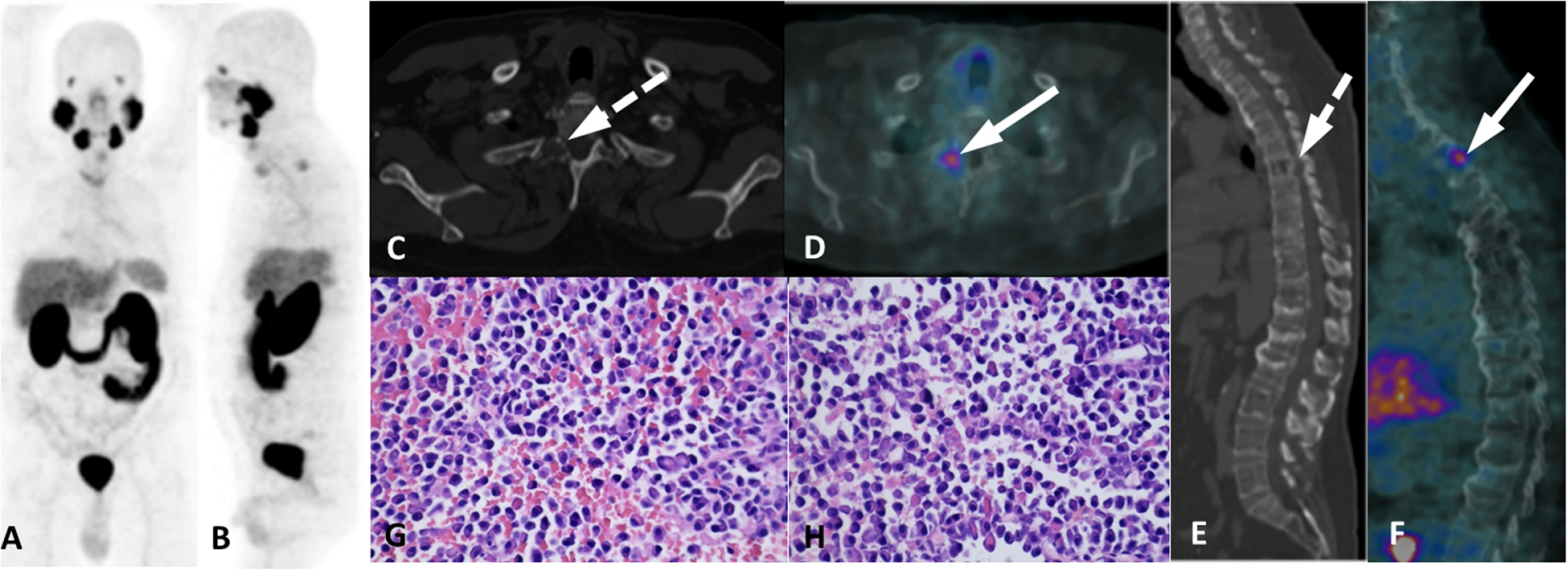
Multiple myeloma with PSMA uptake. ^68^Ga-PSMA PET/CT (a, b), axial CT (c), axial PET/CT (d), sagittal (e) and sagittal PET/CT (f) images show multiple osteolytic lesions (dashed arrows) in the whole axial skeleton but there is a mild uptake in the right pedicle of T2 (arrows), confirmed as multiple myeloma after imaging-guided biopsy (g, h)
